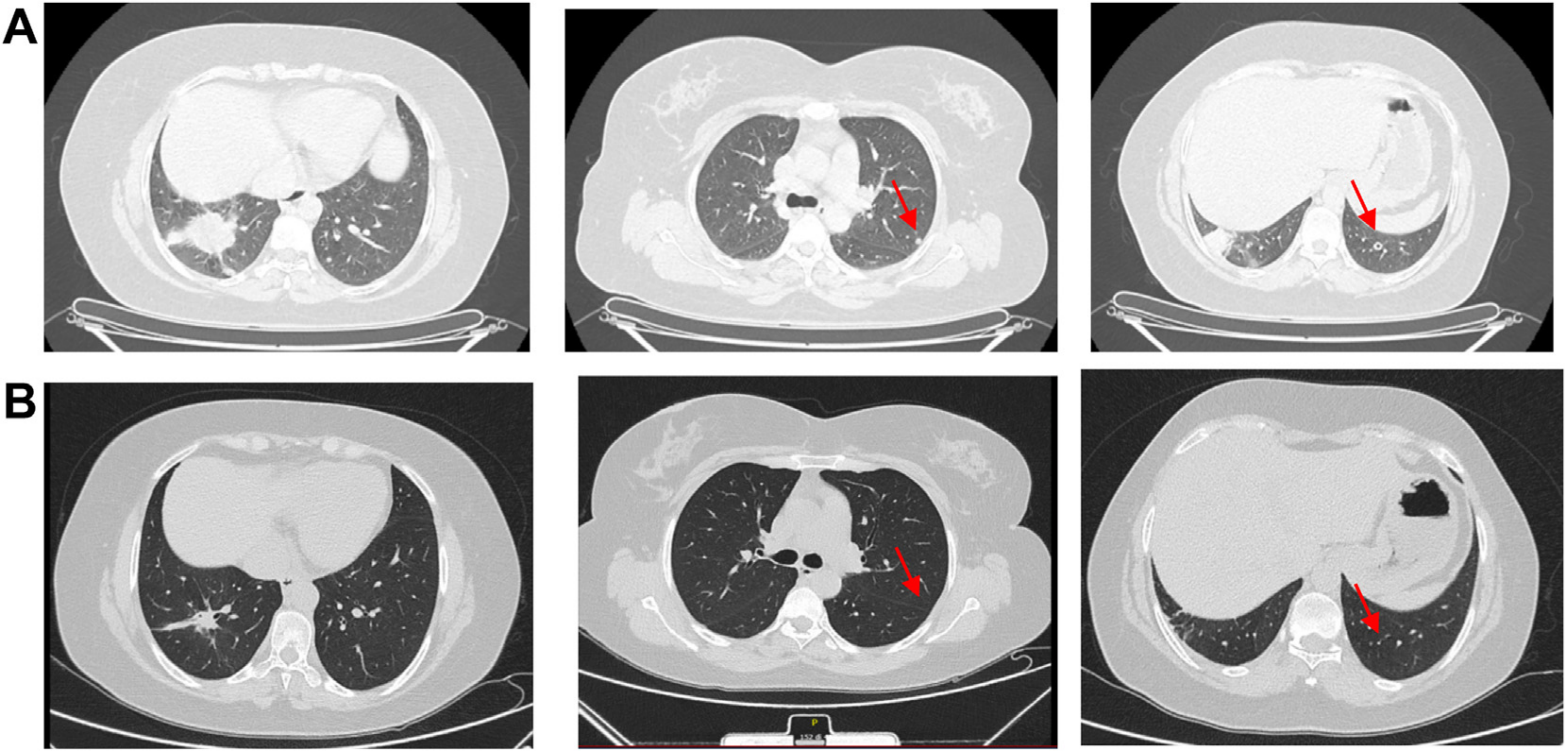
Response to chemoimmunotherapy in a patient with *HER2* mutant NSCLC. Adenocarcinoma of the right lung with metastasis in the mediastinal node and contralateral lung was diagnosed in August 2021. CT scan performed after four courses of cisplatin-pemetrexed-pembrolizumab reported a partial response in the primary lesion and a complete response of all secondary left pulmonary nodules. The response lasted for approximately 24 months *(A, B).* CT, computed tomography.
